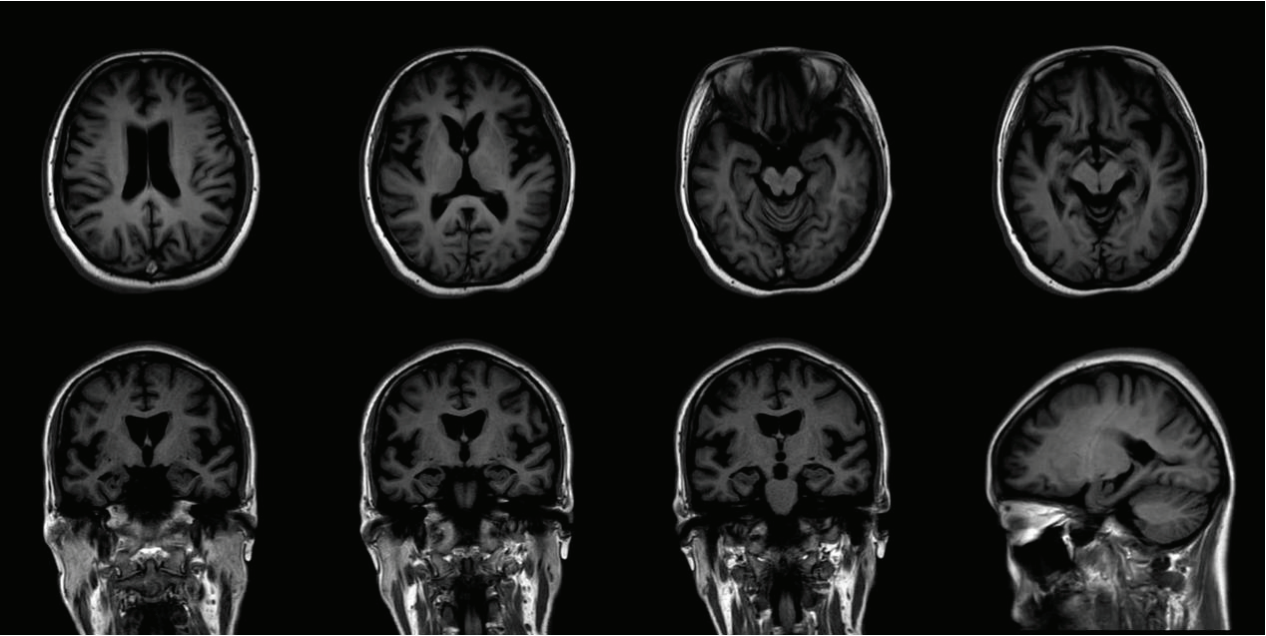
The corresponding MRI scans. MRI revealed diffuse cortical atrophy in the temporal lobe and hippocampal atrophy.
